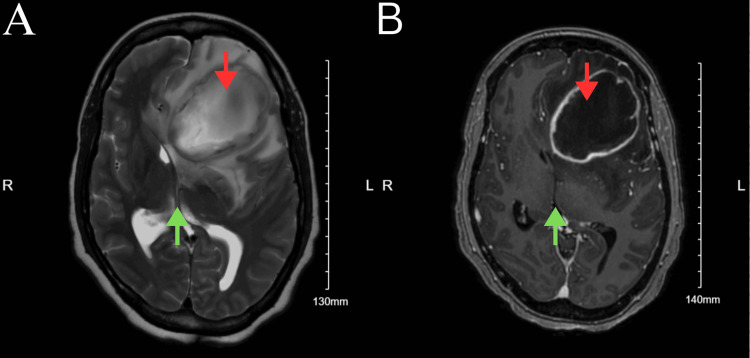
T2-weighted (A) and fluid-attenuated inversion recovery (FLAIR) (B) magnetic resonance imaging (MRI) demonstrating a left frontal lobe mass measuring 6.2 × 4.6 × 3.7 centimeters with thick enhancing wall and central non-enhancement (red arrows). Significant surrounding edema with 1.2-centimeter rightward midline shift (green arrows) and effacement of the left frontal horn. (A) T2-weighted magnetic resonance imaging (MRI), (B) fluid-attenuated inversion recovery (FLAIR) magnetic resonance imaging (MRI). Red arrows: Left frontal lobe mass measuring 6.2 × 4.6 × 3.7 centimeters with thick enhancing wall and central non-enhancement effacement of the left frontal horn. Green arrows: 1.2-centimeter rightward midline shift.
